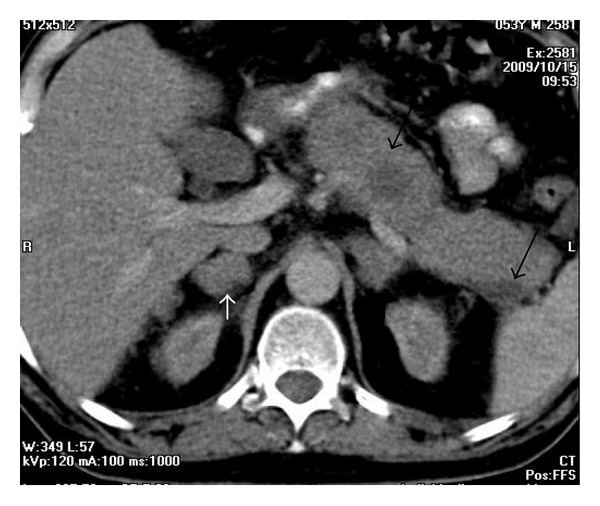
Lymphoma involving pancreas and adrenals. Axial contrast-enhanced CT demonstrates enlarged pancreas with low-attenuation nodules (arrows) and enlarged adrenal glands (small arrows).
